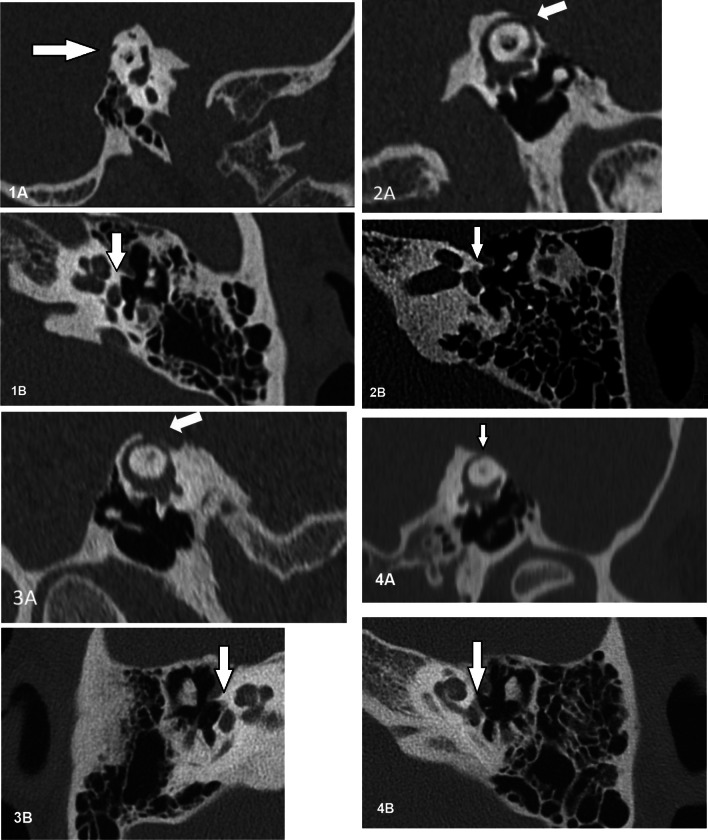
Computed tomography imaging of the mastoid bone in all patients. 1A Case one, Pöschl view showing a dehiscence of the left superior semicircular canal. 1B Case one, axial view of the left temporal bone showing the fissula ante fenestram. 2A Case two, Pöschl view showing a dehiscence of the left superior semicircular canal. 2B Case two, axial view of the left temporal bone showing fenestral otosclerosis. 3A Case three, Pöschl view showing a dehiscence of the right superior semicircular canal. 3B Case three, axial view of the right temporal bone revealing fenestral otosclerosis. 4A Case four, Pöschl view showing a dehiscence of the left superior semicircular canal. 4B Case four, axial view of the left temporal bone showing fenestral otosclerosis
